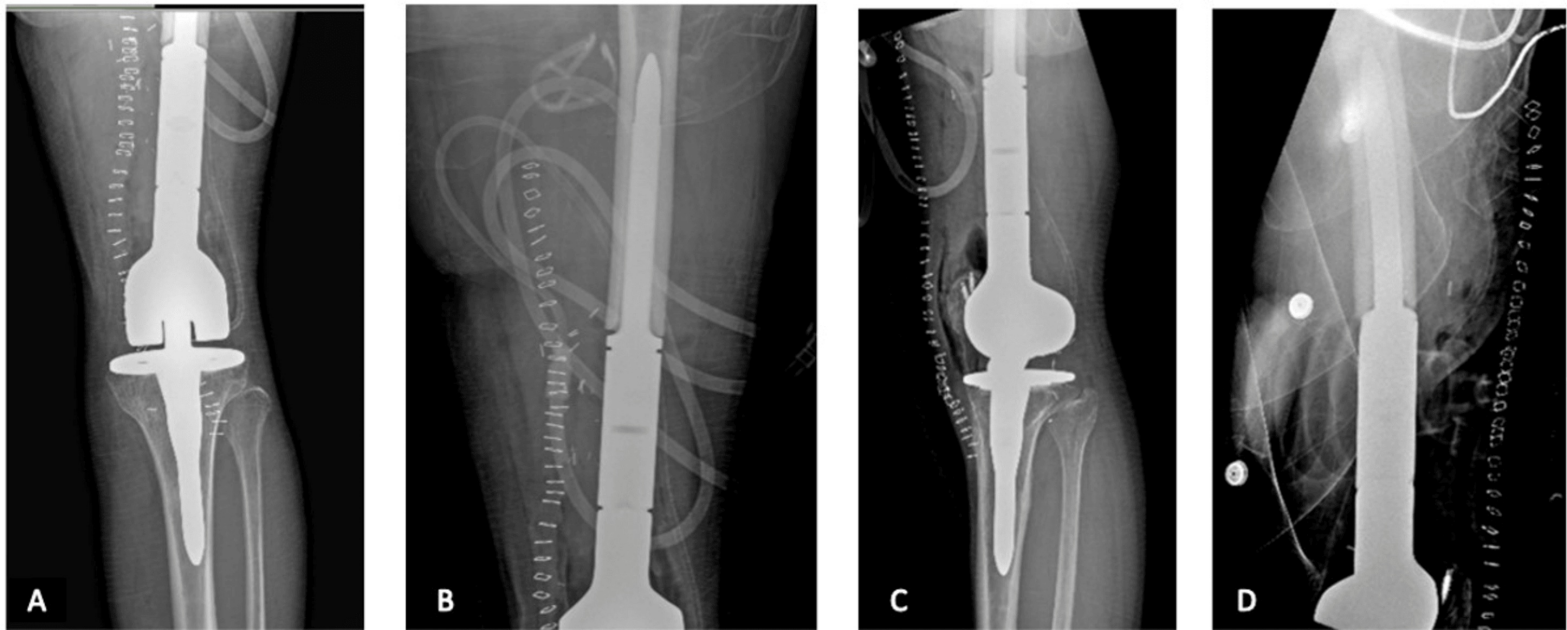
Postoperative X-ray images of the patient. (A-B) Anterior-posterior views of the left knee and femur respectively. (C-D) Lateral views of the left knee and femur respectively.
